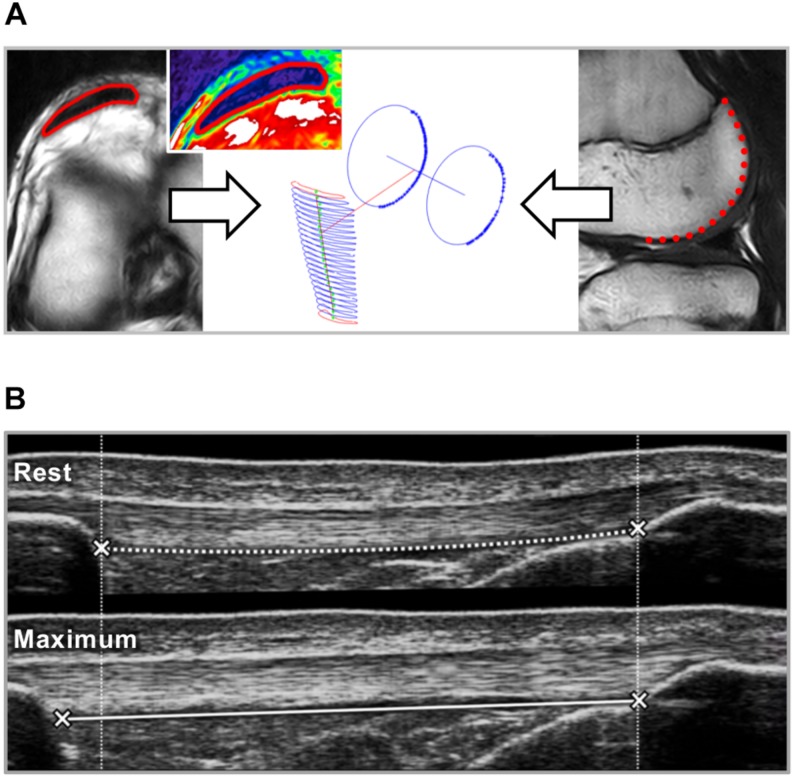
(A) Patellar tendon cross-sectional area (CSA) and moment arm were determined based on magnetic resonance images. In transverse plane images the tendon CSA (red) was segmented over the full length of the tendon (left; inset picture shows the tendon in NIH color scale). Sagittal plane segmentations of the posterior contours of the lateral and medial femur condyles were approximated using a circular fit and the line connecting the centers of the circles was defined as rotation axis (Churchill et al., 1998). The perpendicular distance to the tendon line of action represents the tendon moment arm. (B) The elongation of the patellar tendon during isometric ramp contractions from rest to maximum was measured using ultrasound. Tendon strain, stiffness and elastic modulus were calculated based on the force-elongation and stress-strain relationship between 50 and 80% of the maximum tendon force or stress.
